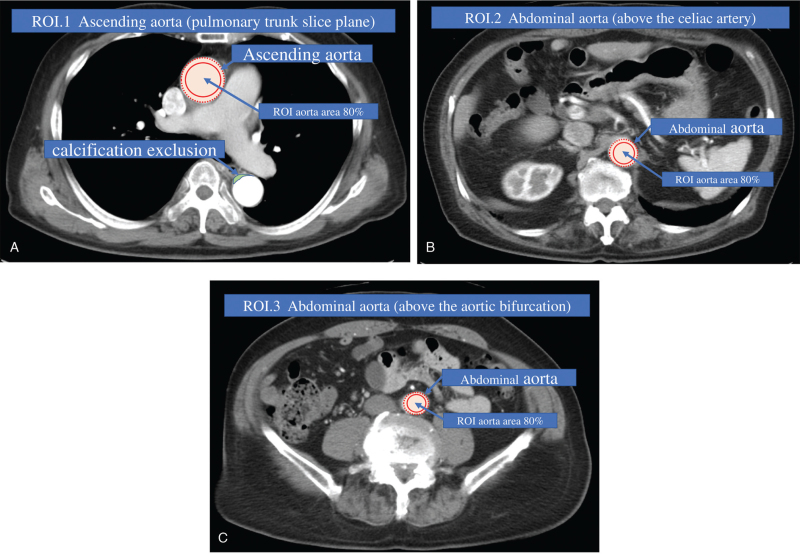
Sites for regions of interest. Aortic CTV is defined as the average of the value taken from 3 aorta sites: at the level of the pulmonary artery bifurcation; just above the level of the celiac artery; and at the level of the bifurcation of the common iliac artery. Region of interest (ROI) in measurement is defined as 80% of the arterial area. CTV = computed tomography value.
